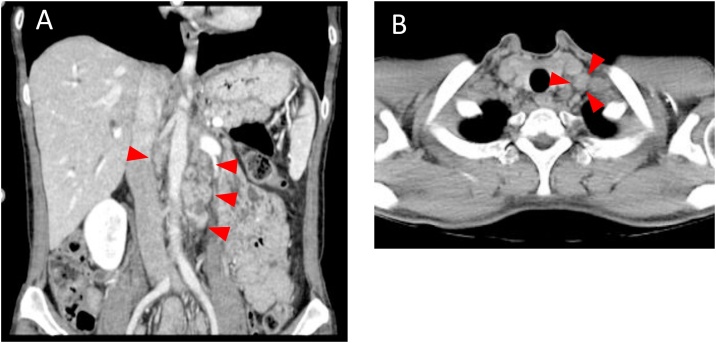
Computed tomography (CT) scan showing swellings of distant lymph nodes (red arrow heads). A: para-aortic lymph nodes (red arrow heads); B: left supraclavicular node (red arrow heads).
